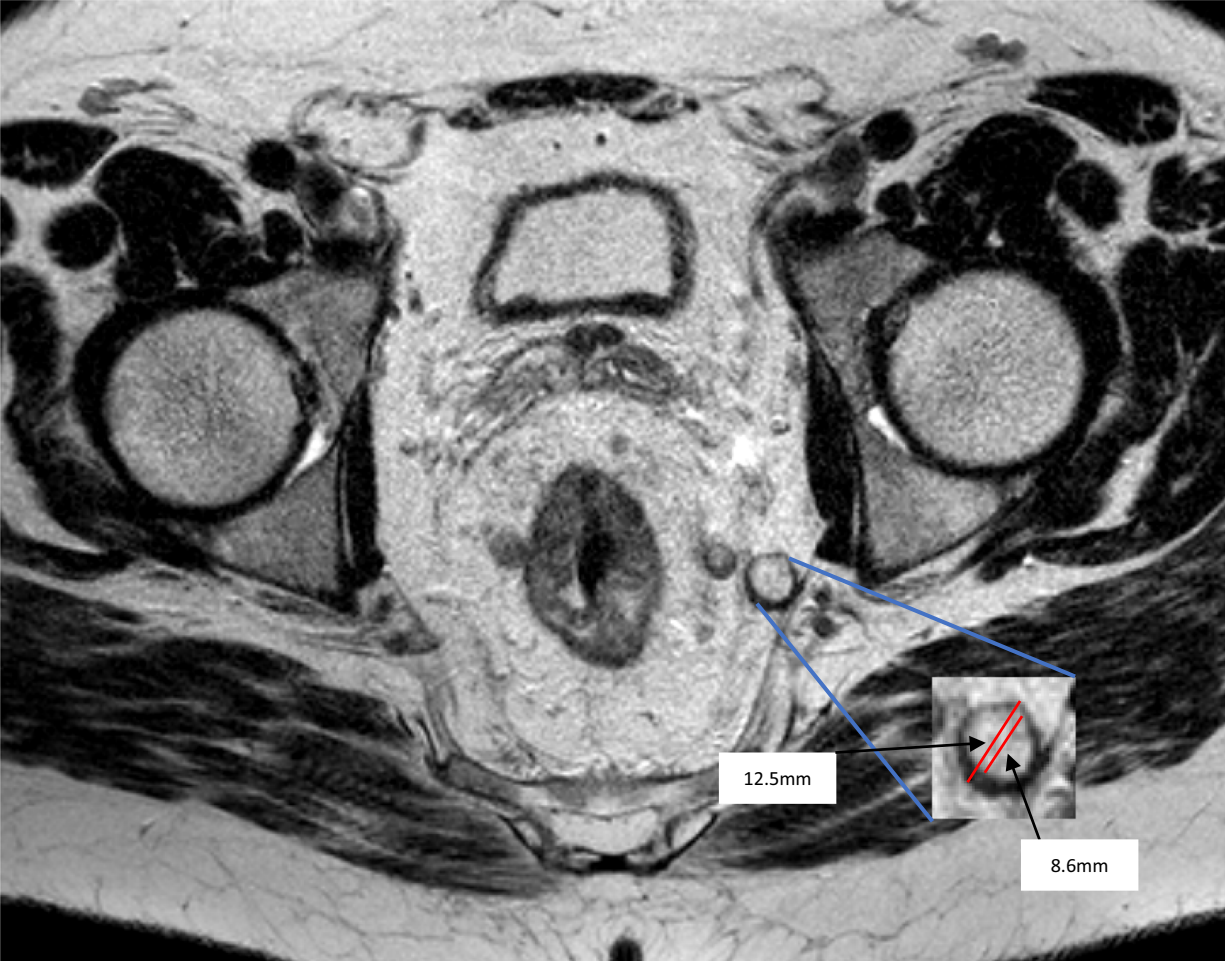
Variation between two potential measurements of the largest LLN from case 2. Axial T2-MRI with a zoomed-in image of the largest LLN from case 2. Two measurements display the variation possible for this LLN due to the presence on a thick outer shell
